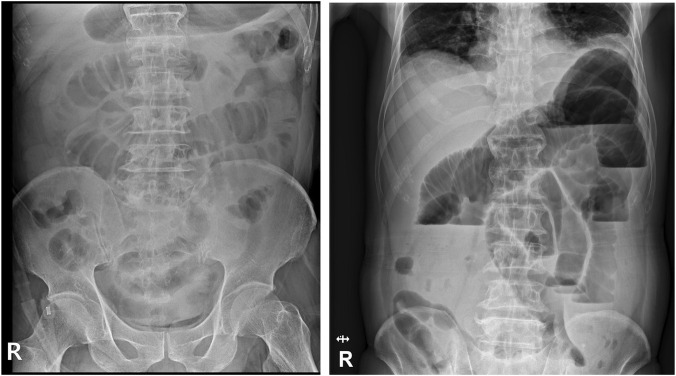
Abdominal radiographs obtained on hospital day (HD) 1 and HD 13 showing progressive small bowel dilatation with multiple air–fluid levels, consistent with worsening ileus.
